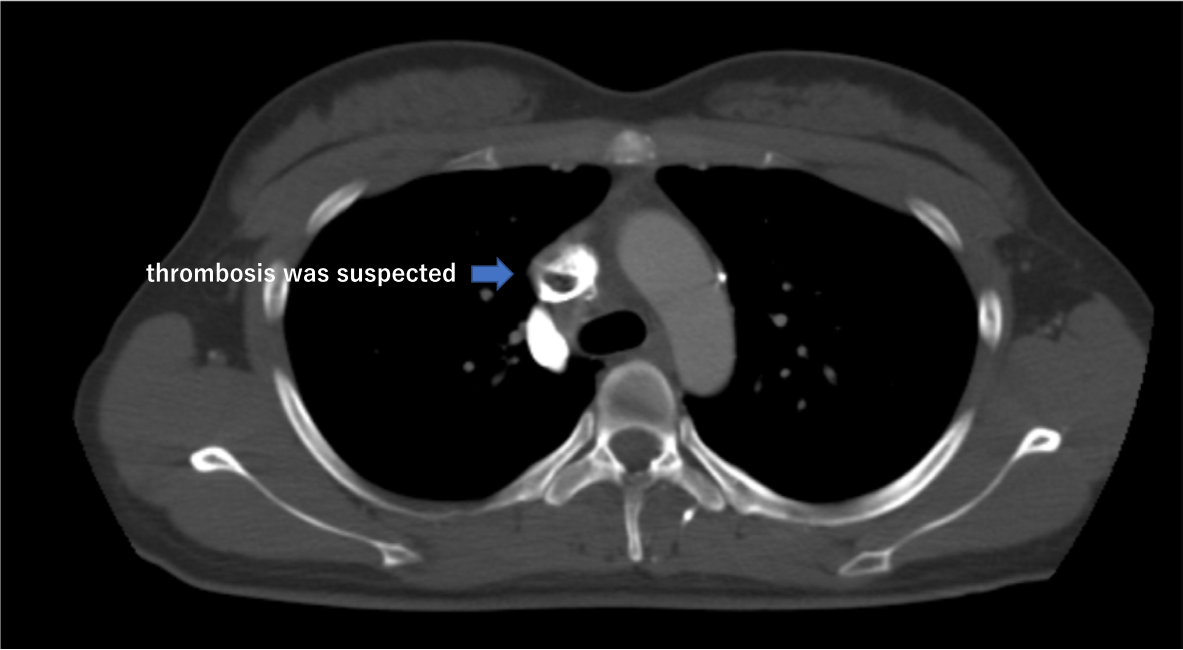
Preoperative CT scan indicating the thrombosis in the SVC
